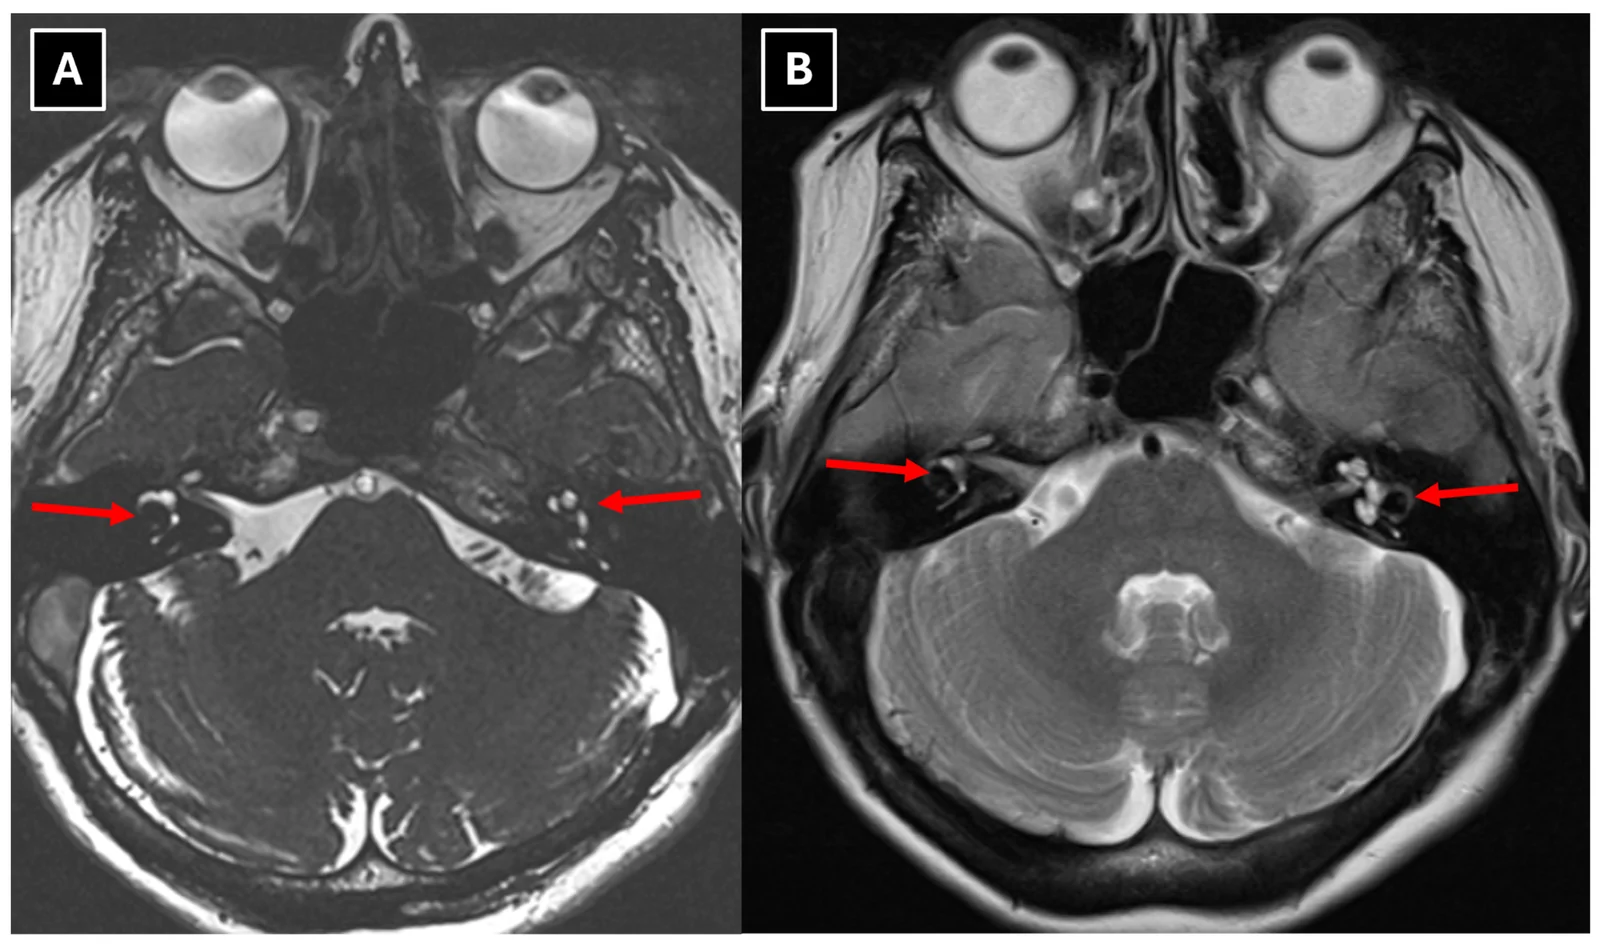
Brain MRI of bilateral otosclerosis in a 59-year-old woman. (A): Axial 3D T2-weighted Constructive Interference in Steady State (CISS) and (B): T2-weighted Turbo Spin Echo (TSE) images demonstrated preserved fluid signal within the cochlea, vestibules, and visualized semicircular canals, without evidence of labyrinthine obliteration. No corresponding T2-weighted abnormalities within the otic capsule can be seen. Red arrow—otic capsule. These T2-weighted sequences are of limited sensitivity for directly depicting otospongiotic changes of the otic capsule. However, they are useful for assessing labyrinthine morphology and excluding alternative retrocochlear pathology. Although the literature indicates that MRI findings in otosclerosis are less widely recognized [5], many authors emphasize their increasing importance in the evaluation of skull-base bone and inner ear pathology, with the T2 CISS sequence being particularly valuable because of its high contrast resolution [6]. MRI provides a better opportunity to assess the size of the cochlear canal, which may in turn be important for treatment planning and for predicting the outcome of surgical treatment [2].
